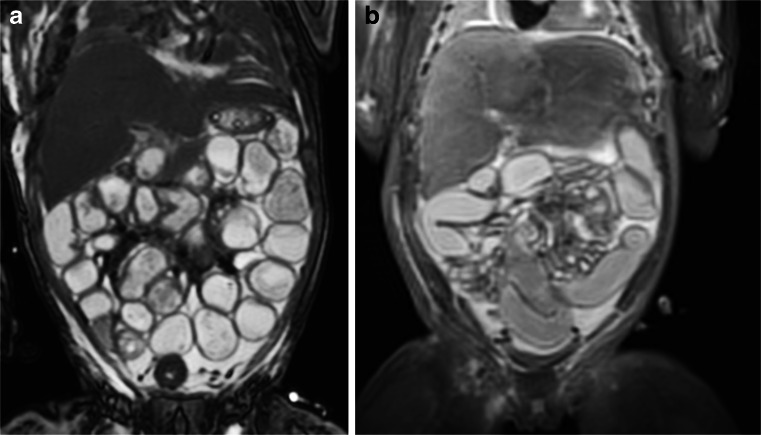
Bowel dilatation. Two examples of coronal T2-weighted postmortem magnetic resonance imaging images from neonatal deaths, which show different degrees of bowel dilatation (left a more severe than right b), which were interpreted as being bowel obstructions. In both cases, these represent normal postmortem dilatation of the bowel, and the bowel was normal at autopsy
